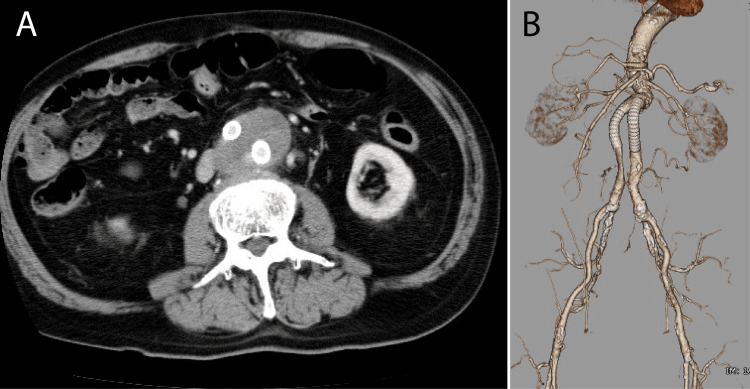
Contrast computed tomography image taken 32 years after artificial blood vessel replacement surgery in which a covered stent was placed inside the artificial blood vessel. (A) An artificial vascular leg was identified in the abdominal aorta sac, and a covered stent was implanted. (B) Volume rendering image. The abdominal aorta had been replaced by an artificial covered stent.
